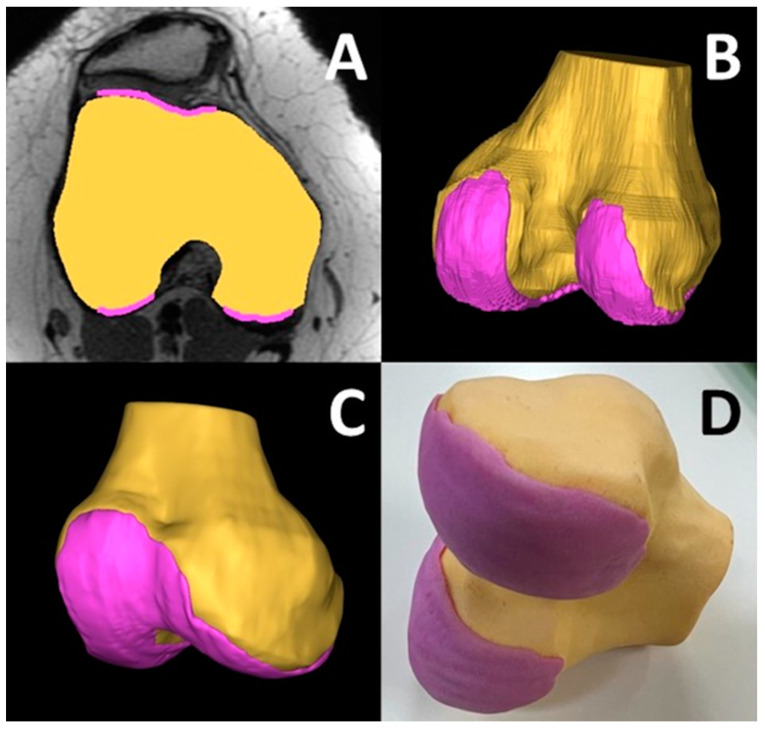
MJT 3D printed distal femur model for three-dimensional visualization of the abnormal anatomy of the trochlear groove for a 20-year old patient. (A) Segmented cartilage (magenta) and distal femur (yellow) masks overlaid on axial cube MRI. (B) 3D visualization of the segmented masks, (C) smoothed surface mesh generated from the masks showing the trochlear groove (D) the 3D printed model showing the cartilage and femur. The degree of abnormality often determines the severity of patellar instability. Three-dimensional printed distal femur can potentially outperform CT and MRI for operative planning.
